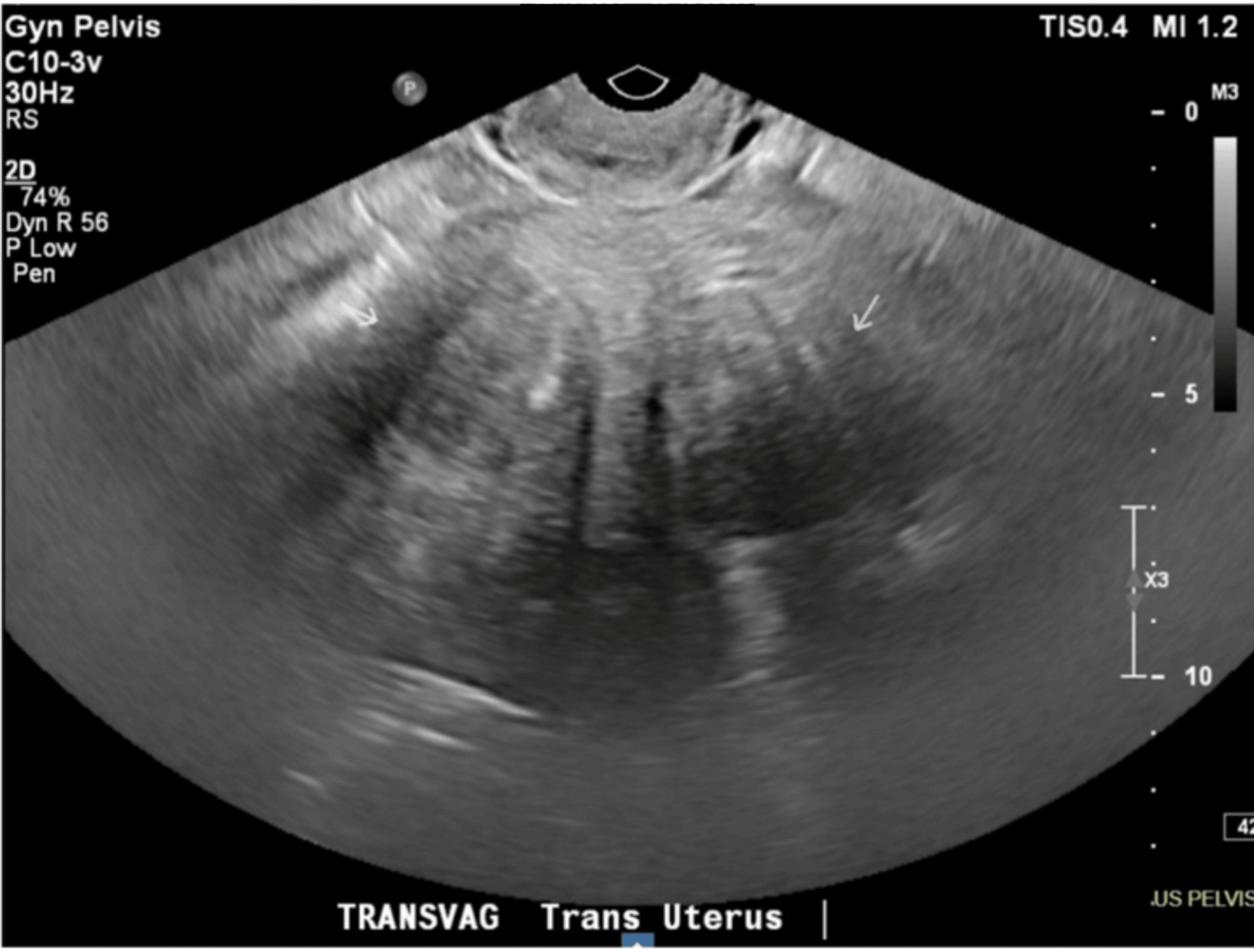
Non-obstetric transvaginal ultrasound showing multiple (at least two) heterogeneously isoechoic shadowing intramural fibroids, indicated by arrows, as follows: left uterine body measuring 3.9 × 5.2 × 4.7 cm; right uterine body measuring 4.2 × 3.8 × 4.0 cm.
